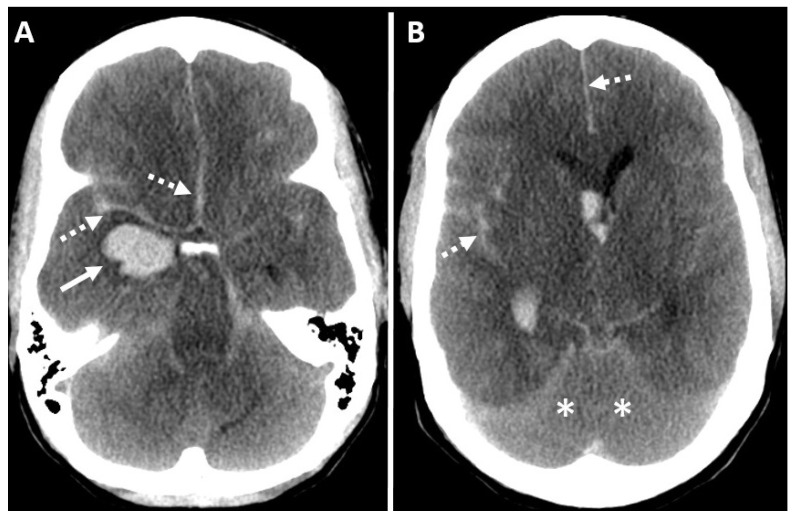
Unenhanced axial CT brain (A) at the level of the temporal lobe and (B) at the level of basal ganglia in a patient with cocaine abuse demonstrate right temporal lobar hemorrhage (solid white arrow in (A)), diffuse subarachnoid hemorrhage (dashed white arrows in (A,B)), and intraventricular hemorrhage. Supratentorial parenchymal hypodensity with hyperdense appearance of the cerebellum (white stars in (B)) called “white cerebellum” or “cerebellar reversal sign”.
